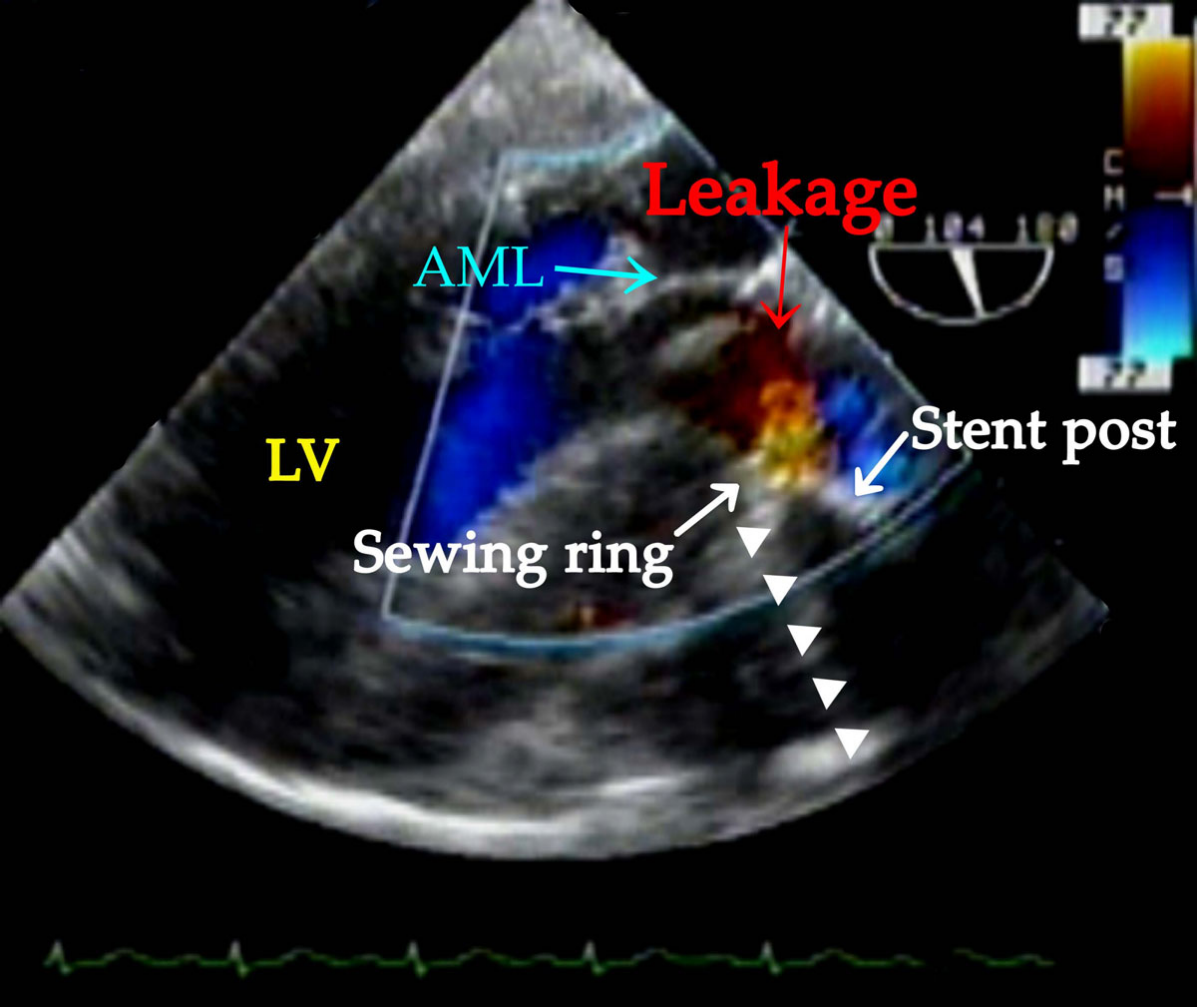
Transesophageal echocardiography showing leakage between the stent-post and the sewing ring before administration of protamine. Trans-gastric long axis view shows the diastolic oblique regurgitant flow toward the anterior mitral leaflet (red arrow) between the stent-post (white arrow) and the sewing ring (white arrow) before administration of protamine and immediately after CPB. The acoustic shadow (triangle) is accompanied by the stent-post and the sewing ring. LV: left ventricle; AML: anterior mitral leaflet.
